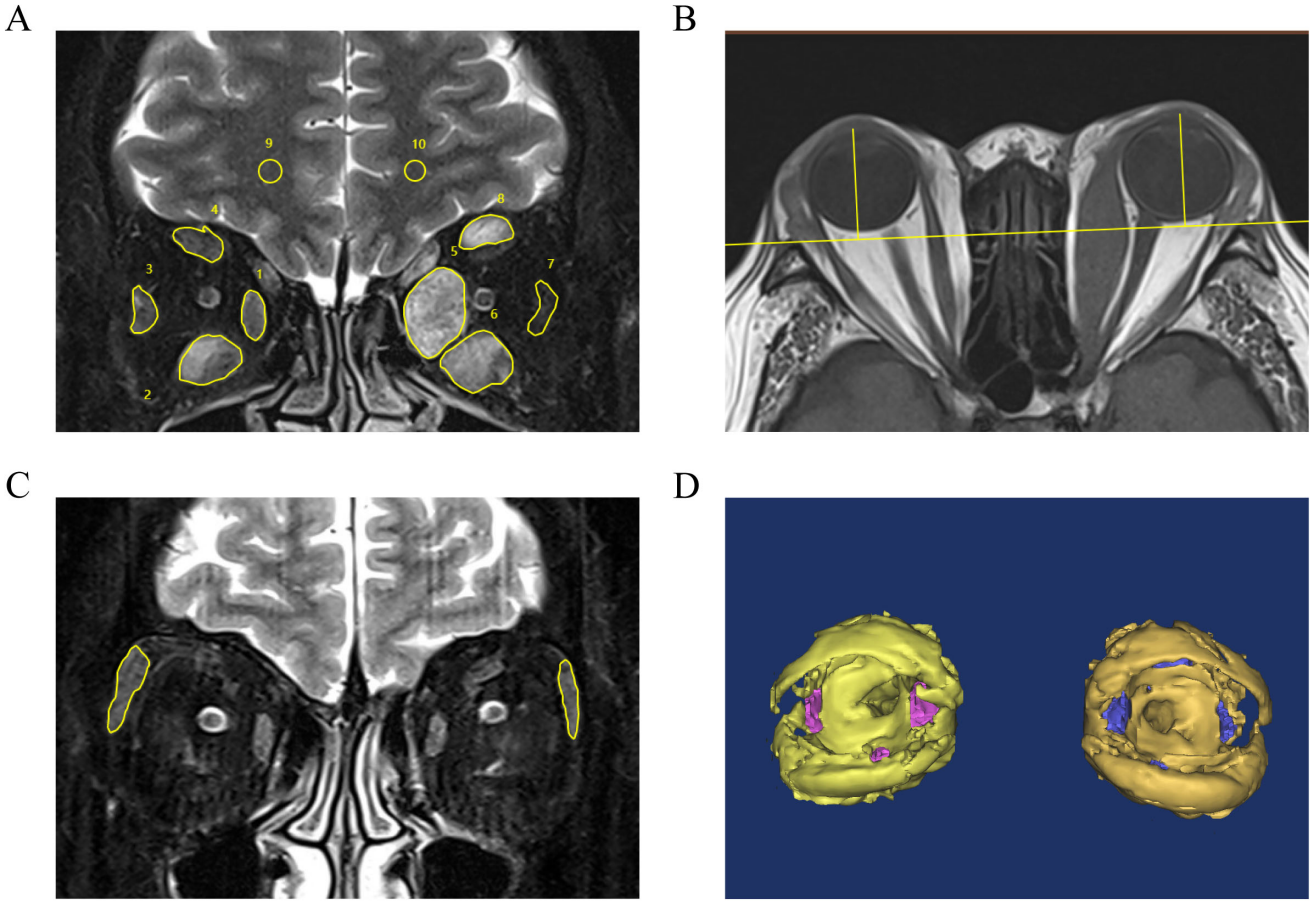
Orbital MRI acquisition and quantitative measurements. (A) Manual segmentation of EOMs and cerebral white matter (reference region) by radiologists. The most severe inflammation was identified in images taken 3, 6, and 9 mm posterior to the globe, and EOMs were outlined to measure signal intensity. SIR max was calculated as the highest value among (SI _EOMs_/SI _white matter_) for individual muscles. The SIRs of the left internal rectus, superior rectus, external rectus, and inferior rectus were 2.56, 2.42, 0.53, and 1.96, respectively; thus, the SIR max=2.56. (B) On the section that bisects the lens, the perpendicular distance from the corneal apex to the line connecting the bilateral zygomatic processes was defined as proptosis (left eye: 24.34 mm; right eye: 24.93 mm). (C) In the coronal series, the image in which the LG appeared the largest was chosen, and the area of the LG was manually enclosed (left: 33.4 mm^2^; right eye: 56.5 mm^2^). (D) Reconstruction of orbital fat and EOM and the volume of the soft tissues were calculated by MIMICs automatically (left eye: EOM volume=9.98 cm^3^, orbital fat volume=22.54 cm^3^). GO, Graves’ orbitopathy; EOMs, extraocular muscles; SIR, signal intensity ratio; LG, lacrimal gland.
